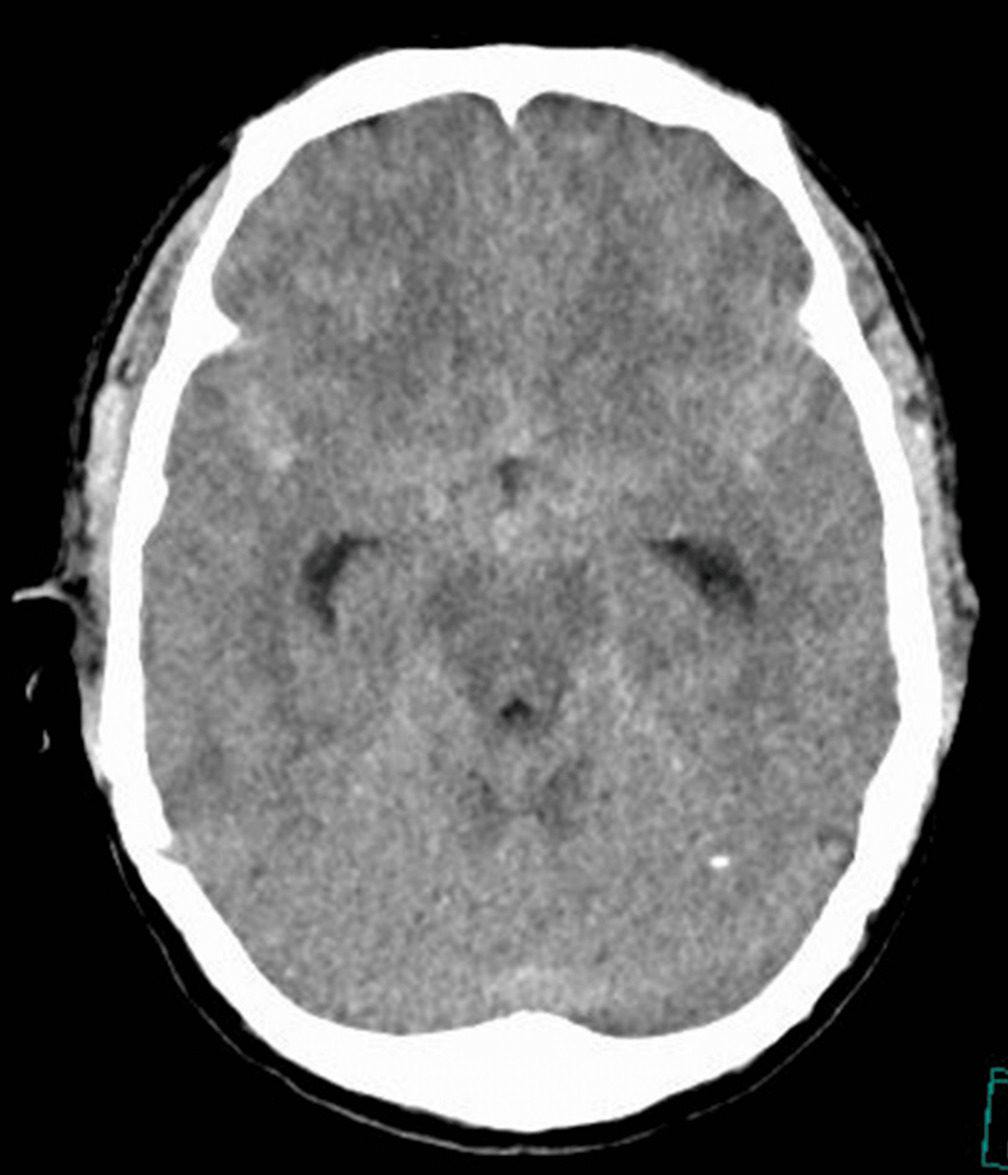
Head CT (Case 2). Non-contrast-enhanced axial head CT showing diffuse subarachnoid hemorrhage involving the basal and ambient cisterns.
